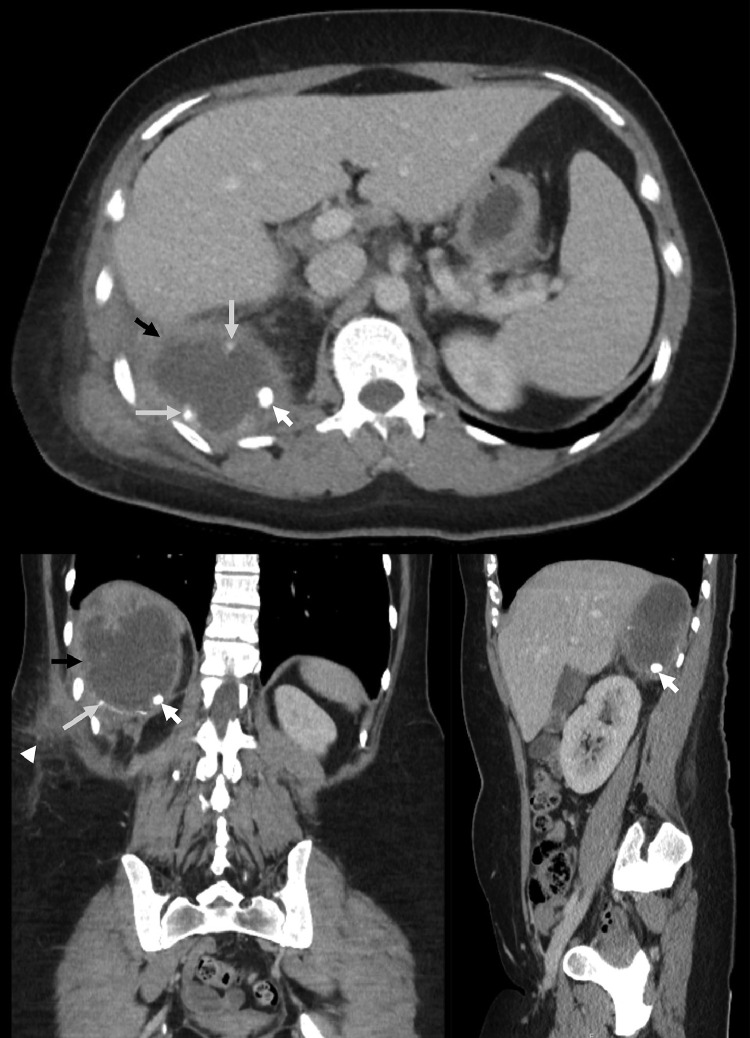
Axial (top), coronal (bottom left) and sagittal (bottom right) CT images showing a 9 cm retro hepatic abscess (black arrows) with a 10 mm appendicolith (white arrows) and an extension into the subcutaneous and intramuscular compartments (white arrowhead). Specks of calcification were also noted in the wall of the abscess cavity (gray arrows).
